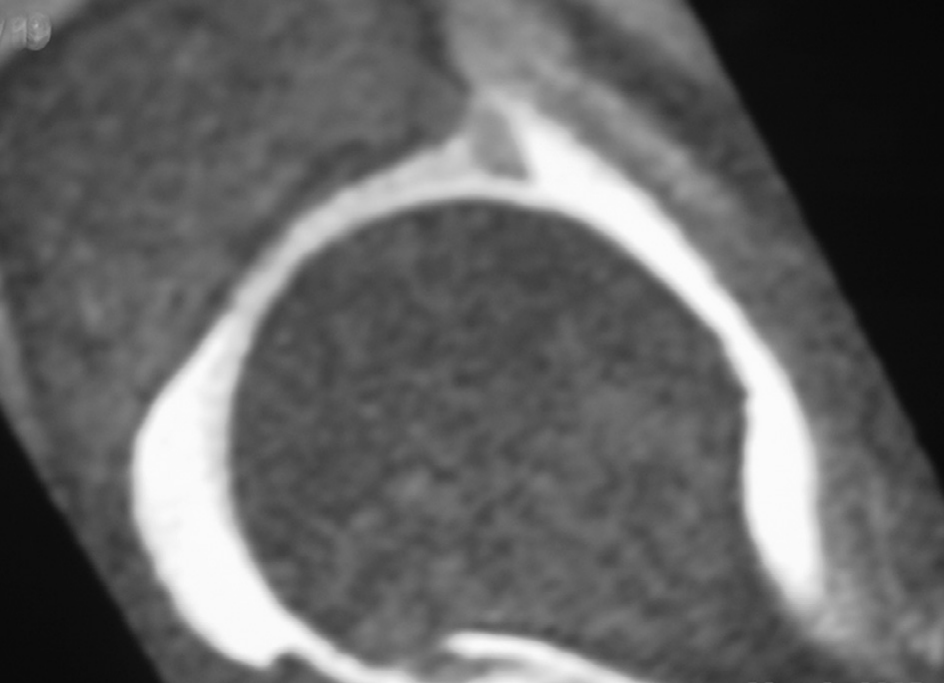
MRI arthrogram of left hip indicating acetabular labral tear.
